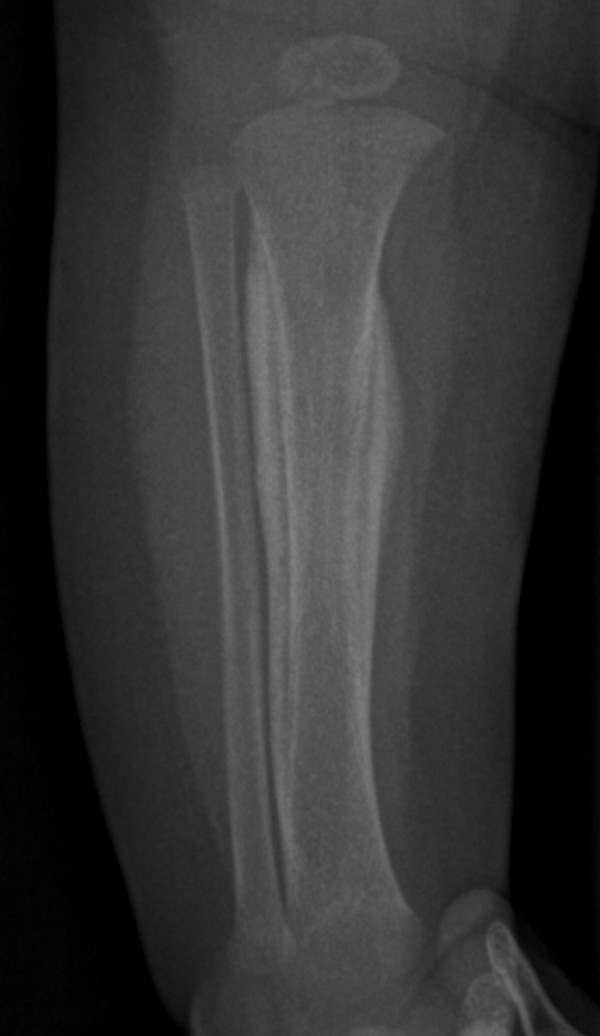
Anteroposterior radiograph of the tibia showed a thick and broad ballooning occupies the diaphyses (proximally and distally) there is gaining in the diameter comes from subperiosteal new bone apposition by intramembraneous bone formation.
